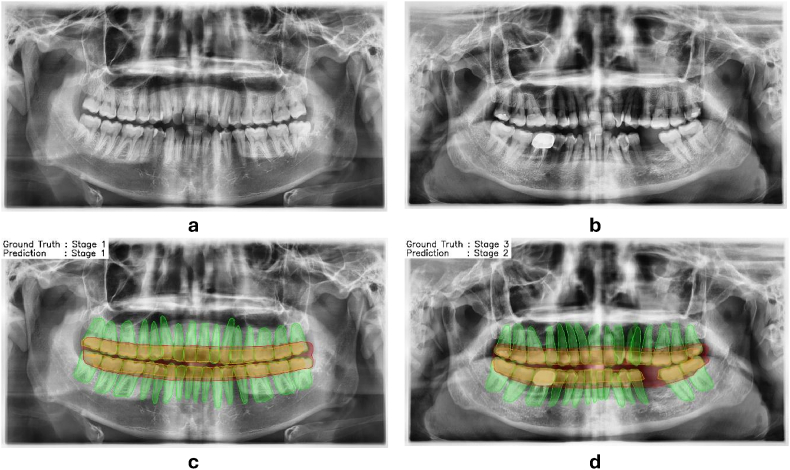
(a) and (b) Panoramic radiographs used for testing the Two-Stage CNN model, with (c) the correct detection results from radiograph (a) and (d) the incorrect detection results from radiograph (b).
